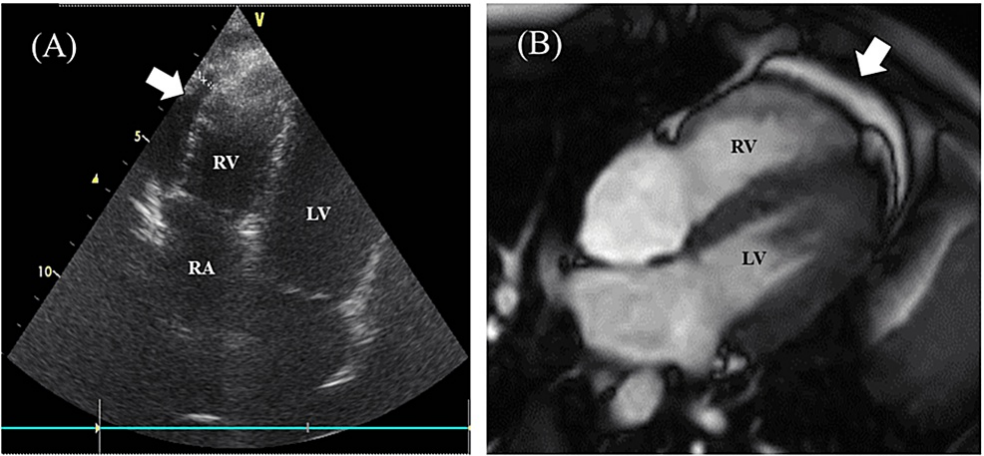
Echocardiogram and cardiac four-chamber cine magnetic resonance image (A) Echocardiogram obtained on the 33rd day after a second COVID-19 messenger RNA vaccination. Pericardial effusion is observed at the right ventricular side. (B) Cardiac four-chamber cine magnetic resonance image obtained on the 46th day after vaccination. Pericardial effusion is observed, but no abnormalities in the myocardium were found RA: right atrium; RV: right ventricle; LV: left ventricle
